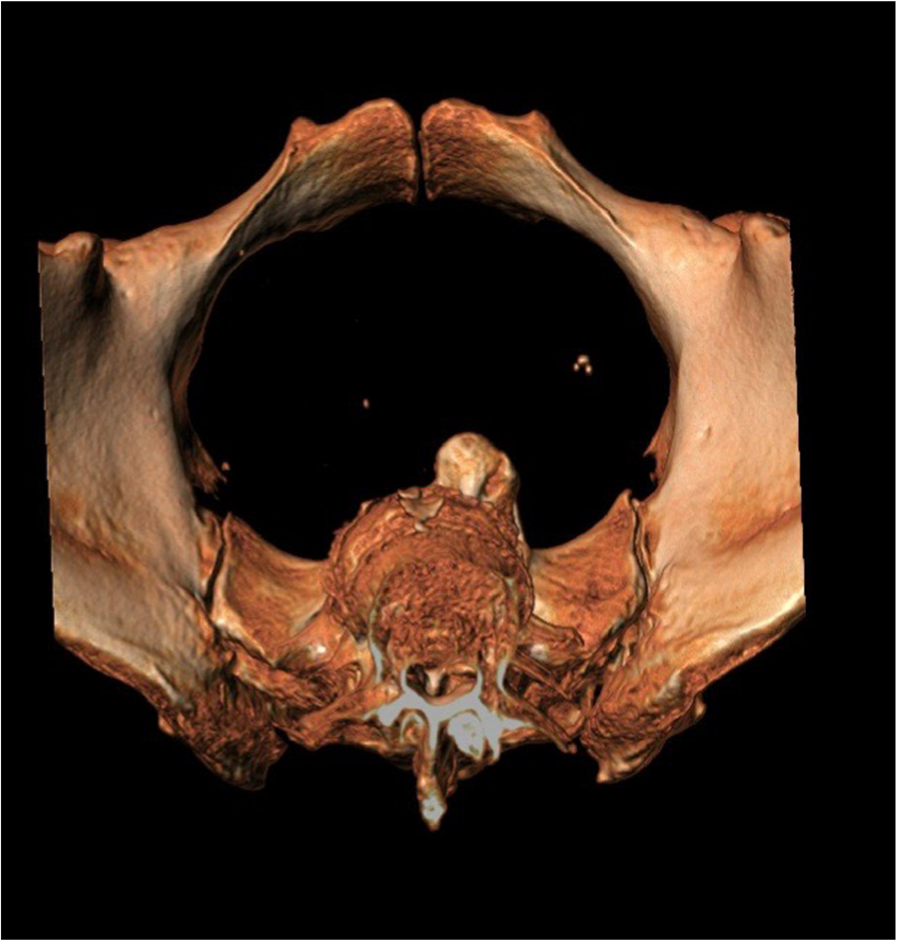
Transverse plane 3D CT scan: sacral promontory
